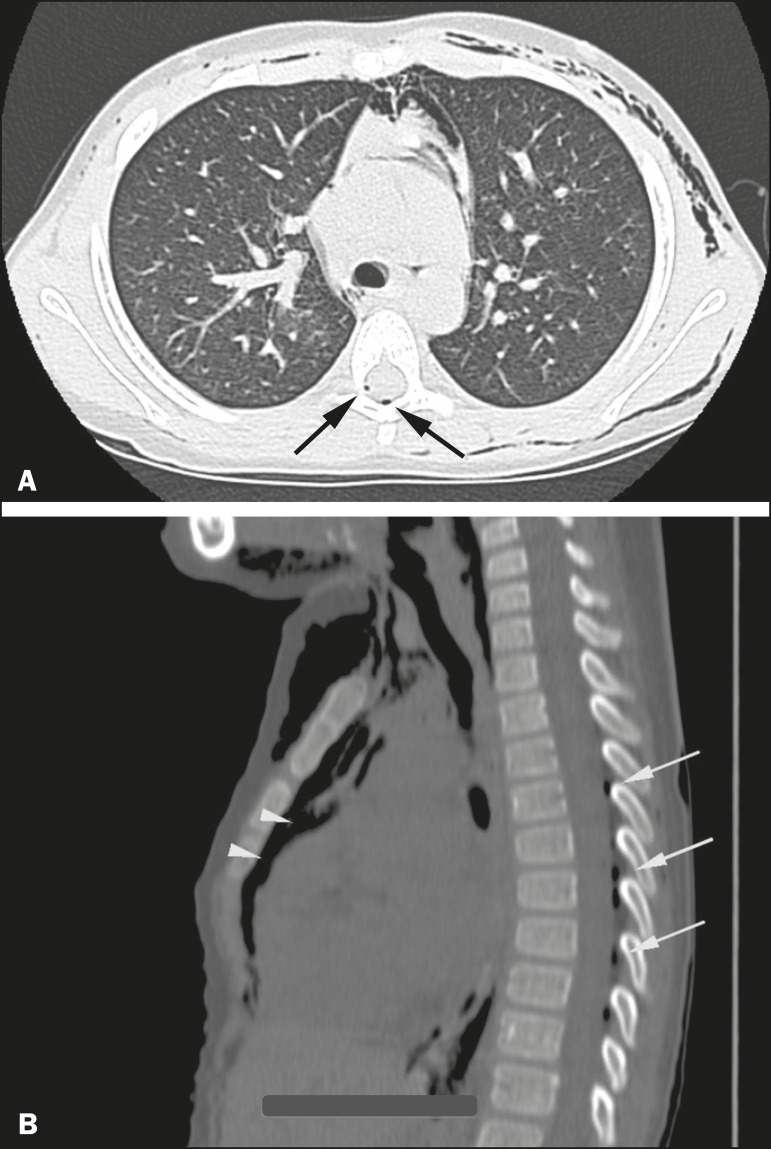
CT of the chest in the axial (A) and sagittal (B) planes showing
